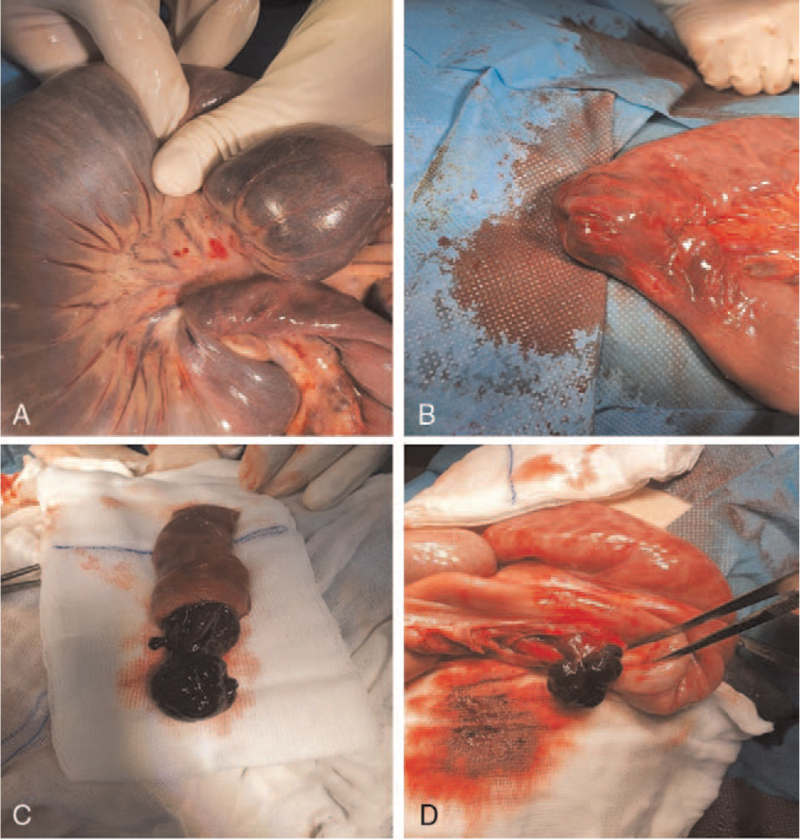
A–D. Reformatted coronal CT scan of the abdomen showing “coffee bean” appearance of the jejunal loop (red arrow) with transition point to duodenal loop (pointed red arrow) (A and C). Axial-contrast-enhanced CT scan of the abdomen showing dilated jejunum loops, filled with fluid (red arrows) with the swirled appearance of mesentery typical for volvulus (pointed red arrow) (B). Reformatted sagittal CT scan showing dilated jejunal loop with mild postcontrast enhancing (red arrow) and swirled mesentery (pointed red arrow) (D). CT = computed tomography.
